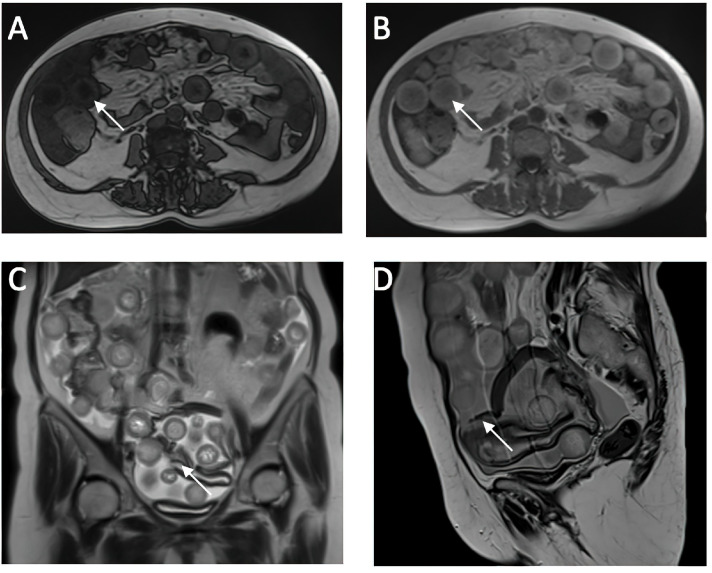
Cross-Sectional MRI Analysis of Abdominal and Pelvic Organs. (A,B) in-phase and op-phase images showing signal dropout on the op phase, suggesting fat content of the sphere (C,D) ruptured cyst wall which is hypointense on the T2w sequence.
